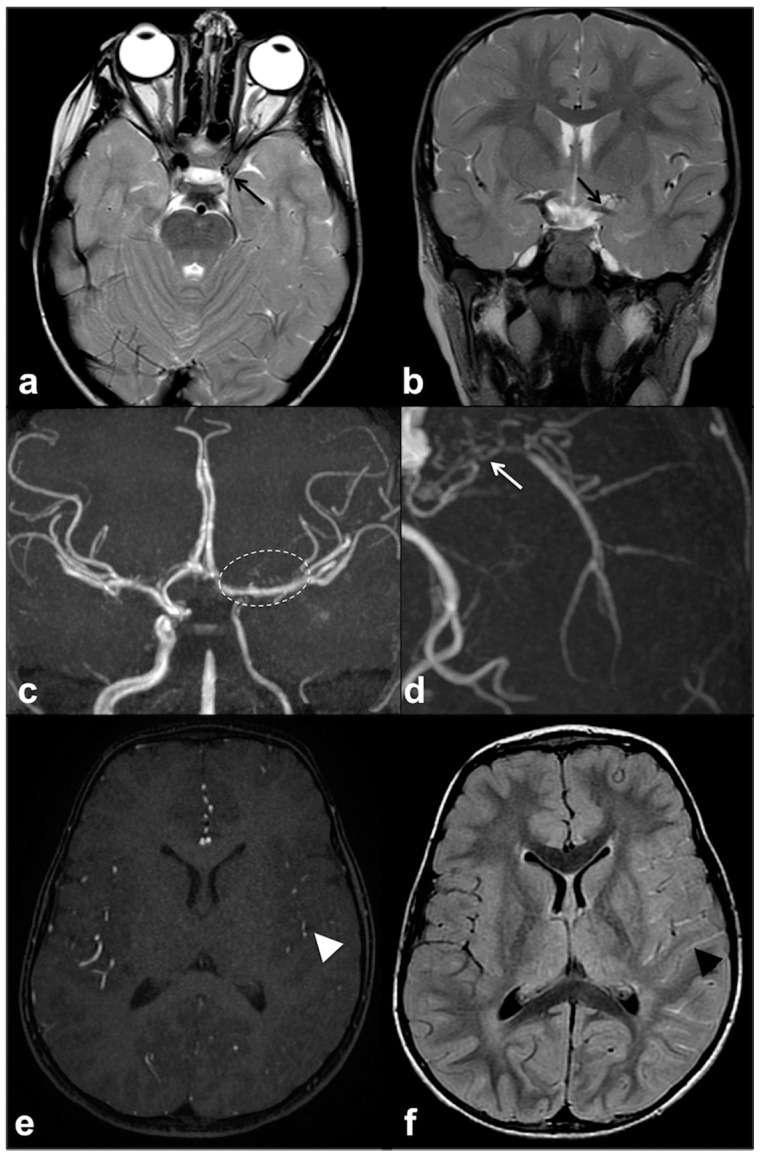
Axial (a) and coronal (b) TSE T2w MRI of an 8 year-old girl with NF1, showing reduced visualization of left cavernous-supraclinoid internal carotid and proximal left middle cerebral arteries’ flow void (black arrows). Time-of-flight 3D MR angiography maximum intensity projection (c) confirmed the severe stenosis of left internal carotid artery and proximal segment of left middle cerebral artery, whereas distal segments are partly supplied by collaterals; dotted line region magnification (d) showed multiple small collateral vessels in proximal left middle cerebral artery region (white arrow). Axial time-of-flight 3D MR angiography (e) confirmed a reduction in distal middle cerebral artery branches flow (white arrowhead), with a diffuse net of prominent leptomeningeal collaterals with high signal on FLAIR (f) due to slow flow (black arrowhead).
